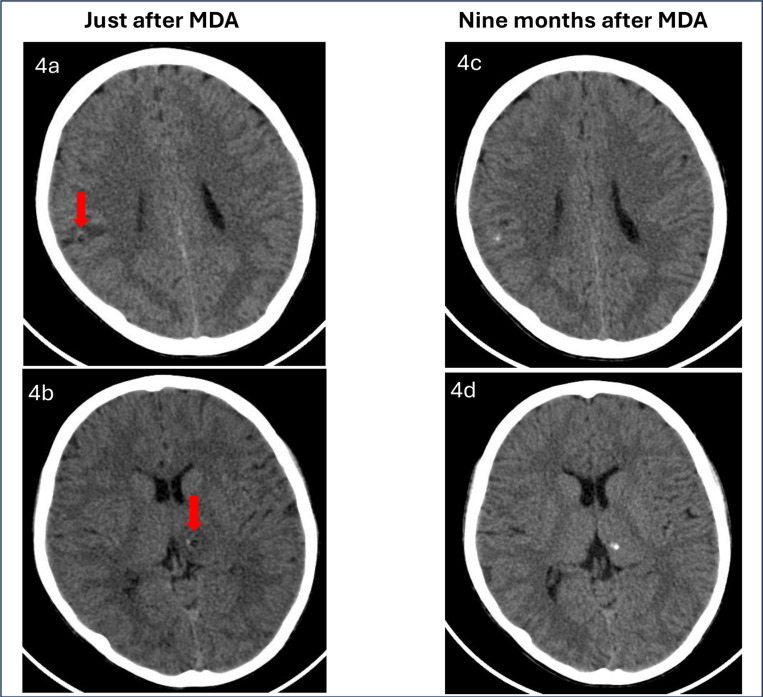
Computed tomography (CT) scan images of the boy who presented seizures after the MDA in 2022. 4a: colloidal stage, 4b: vesicular stage, 4c, d: calcifications.
